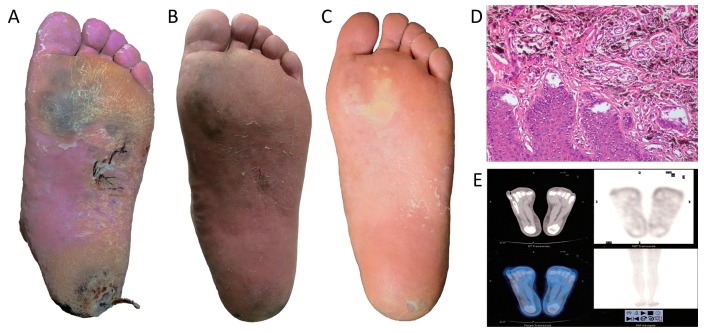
Gross examination of the skin lesions in the patient’s left foot 2 weeks (A), 5 weeks (B) and 24 months (C) after BNCT; (D) Pathological analysis after BNCT; (E) PET/CT scan after BNCT (reprint from Ref. [122]).
